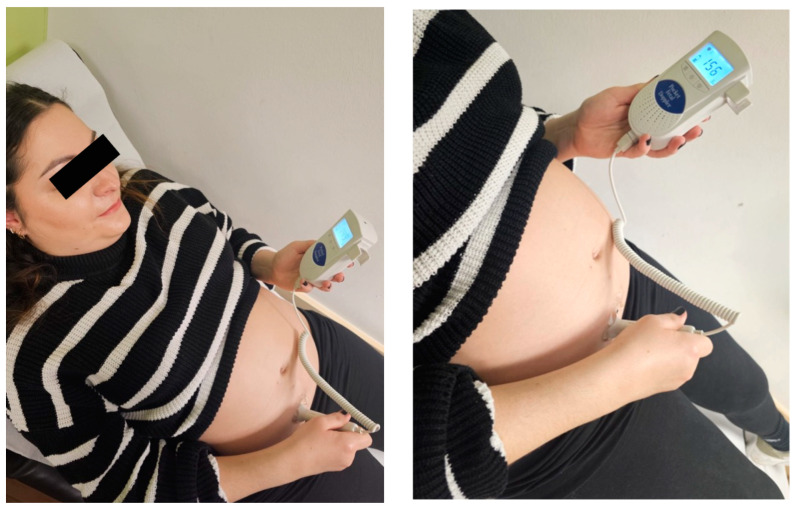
Patient measuring fetal heart rate using handheld Doppler.
